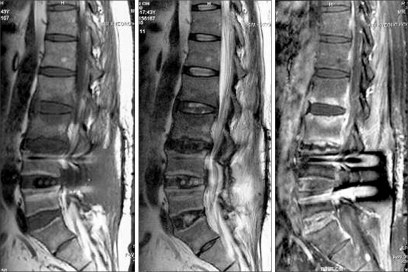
Sagittal T1- and T2-weighted and contrast-enhanced images at 6 months postoperative. Infectious spondylodiscitis was apparent at L2-S1, and anterior epidural abscess formation was apparent at the L2-L3 level.
